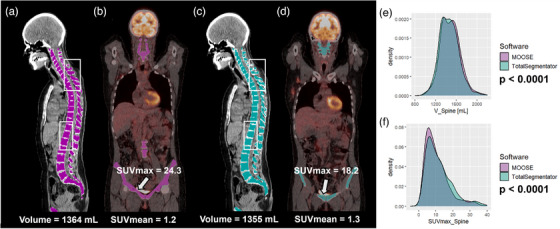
Analysis of the spine and pelvis segmented by MOOSE and TotalSegmentator. (a–d) [18F]FDG PET/CT scans of one patient with spine and pelvis segmented by MOOSE (in purple) and TotalSegmentator (in blue). The white box highlights the mismatch between the segmentations. e–f. Distribution of spine and pelvis volume (in mL) and SUVmax for the two algorithms for all patients.
